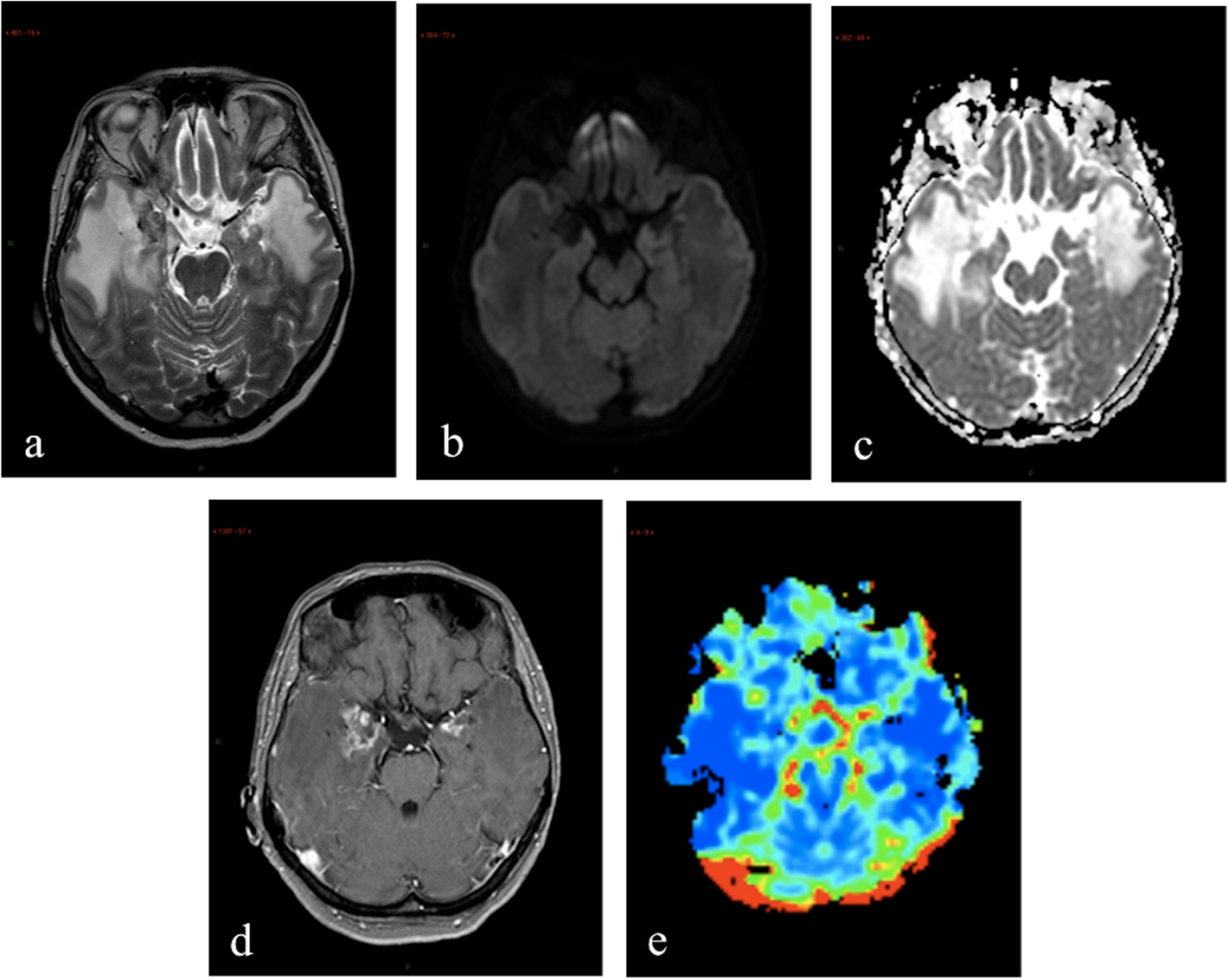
MRI performed 22 months after 78.8 Gy carbon ion therapy in a Patient with malignant peripheral nerve sheath tumor. T2-weighted image (a) shows disomogeneous areas of altered signal, with hypo- and hyperintense components, located in mesial temporal regions both on the right and on the left, and surrounded by extensive edema involving subcortical polar and mesial temporal white matter. DWI documents no diffusion restriction within the enhancing areas with evident hyperintense areas in ADC map on both sides (b, c). Axial post contrast T1-weighted image (d) shows the presence of inhomogeneous contrast-enhancing lesions in mesial temporal regions both on the right and on the left. rCBV map (e) shows hyperperfusion of the left enhancing area and no hyperperfusion of the enhancing area on the right
